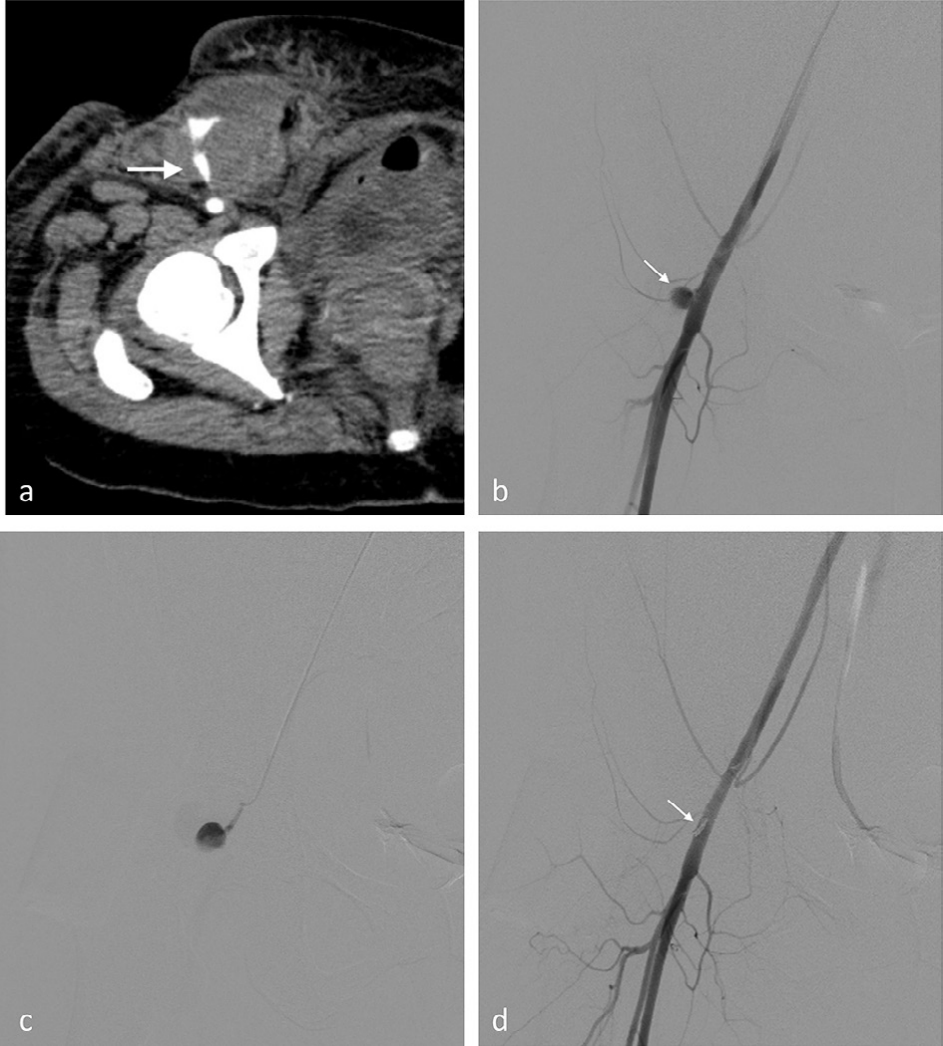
(Case 2) Common femoral pseudoaneurysm after uterine arterial embolisation. (a) CE-CT shows a large haematoma with pseudoaneurysm (arrow) despite the manual inguinal compression. (b) A pseudoaneurysm (arrow) of the common femoral artery is noted on the right femoral arteriogram. (c) The aneurysmal neck is cannulated using a pre-shaped pigtail catheter with cutting of the tip. (d) After embolisation of the aneurysmal neck (arrow), the pseudoaneurysm is successfully treated.
